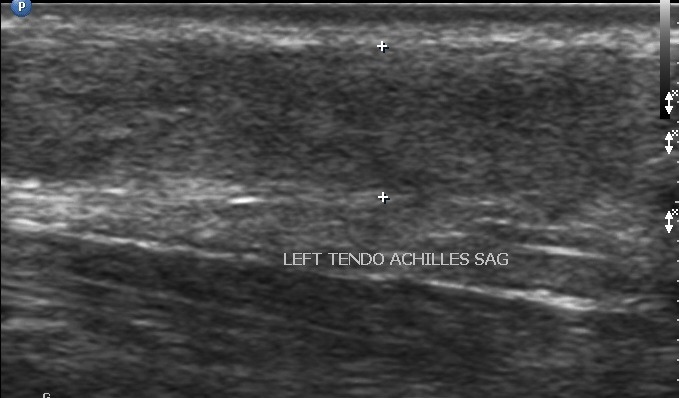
achilles tendinopathy 49 year old female presented with ankle swelling and heel pain for one week. Longitudinal ultrasound of the Achilles tendon shows an enlarged thickened tendon with loss of the normal fibrillary echo-texture
